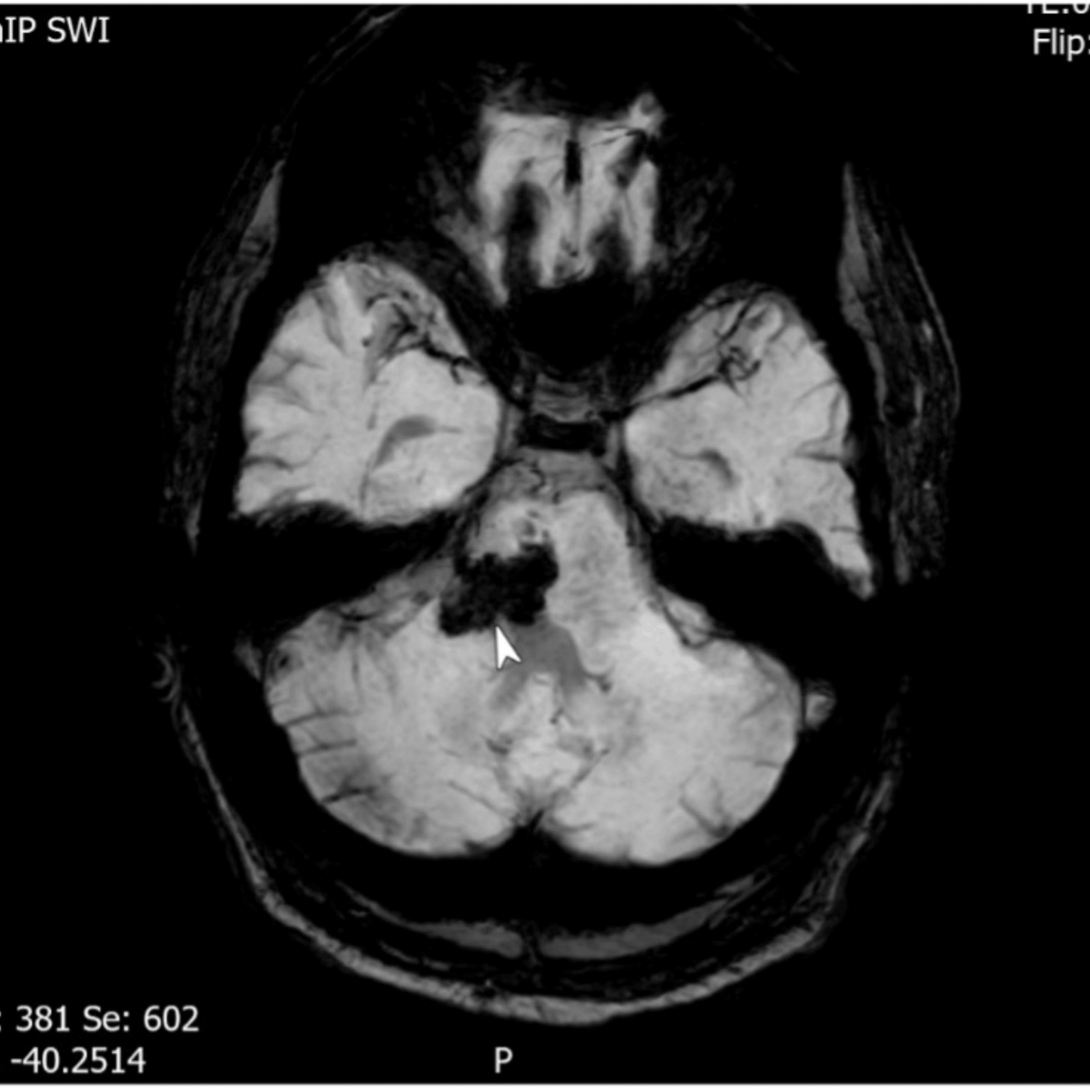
Axial SWI (susceptibility weighted imaging) brain MRI (six weeks after presentation) showing the old right pontine bleed adjacent to the middle cerebellar peduncle
